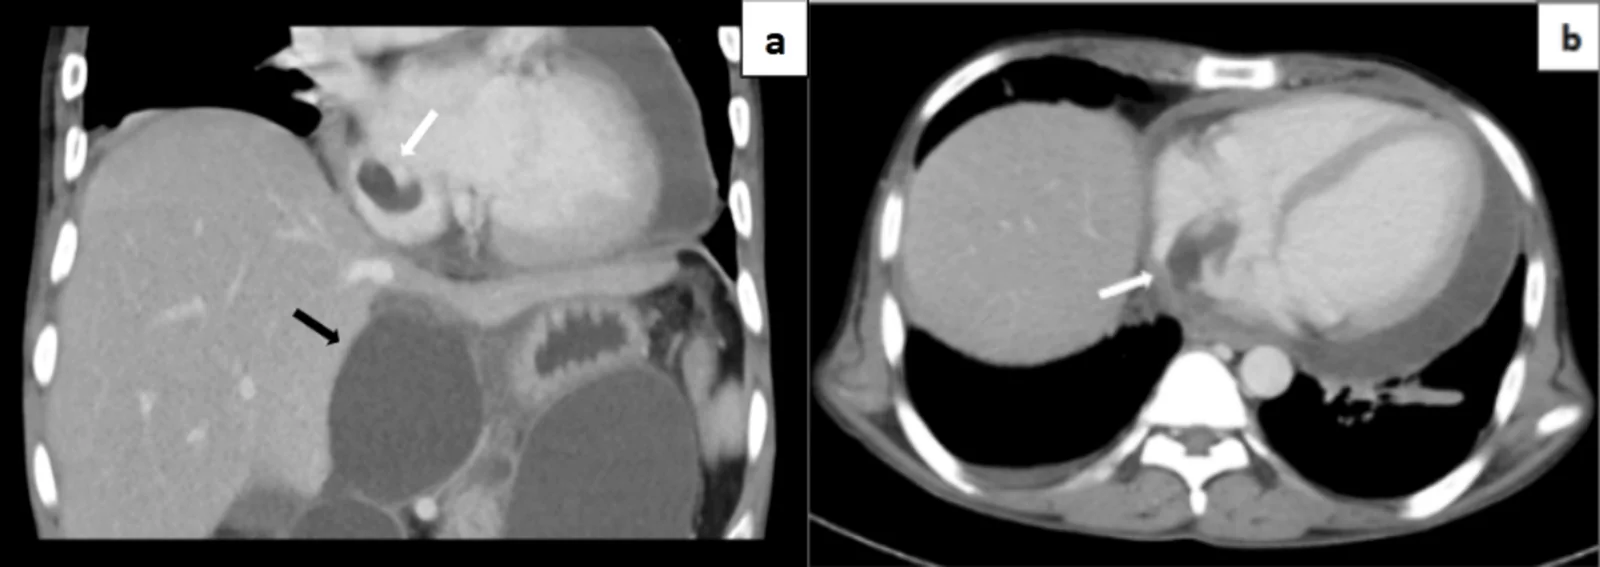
Contrast-enhanced CT of thorax and abdomen (a) Coronal section shows a large, well-defined pseudocyst (black arrow) in the abdomen and a hypodense fluid-attenuating filling defect in the right atrium (white arrow); (b) Axial sections show an irregular hypodense fluid-attenuating filling defect within the right atrial cavity (white arrow)
